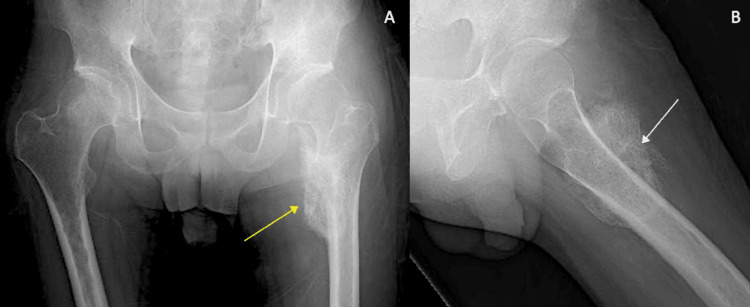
Patient 1 postoperative X-ray Anteroposterior (A) and lateral view (B). The yellow arrow shows the remaining heterotopic ossification post-resection. The white arrow shows the remaining heterotopic ossification post-resection on the surface of the femur.
